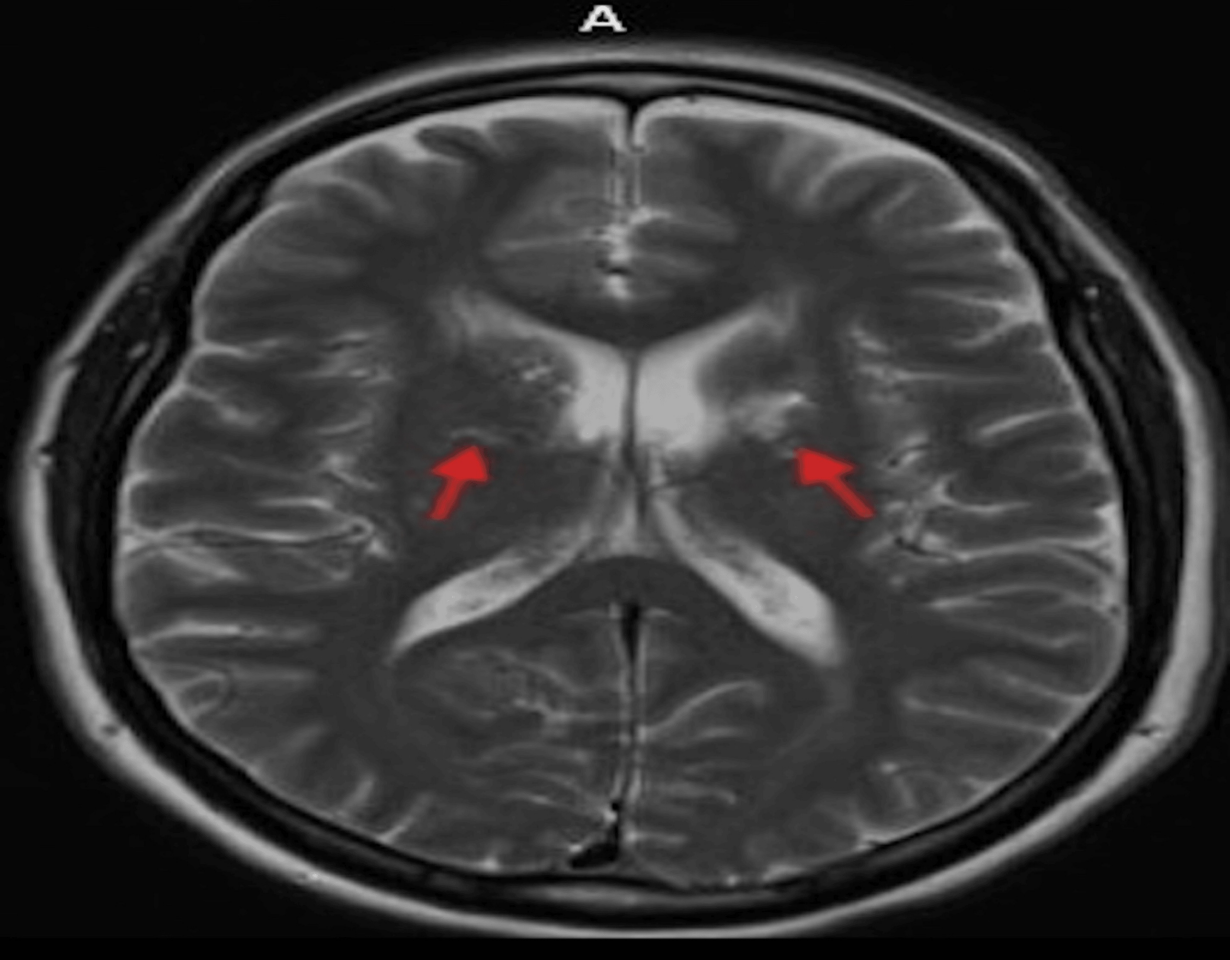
CT head showing chronic bilateral basal ganglia infarcts with microvascular changes.
